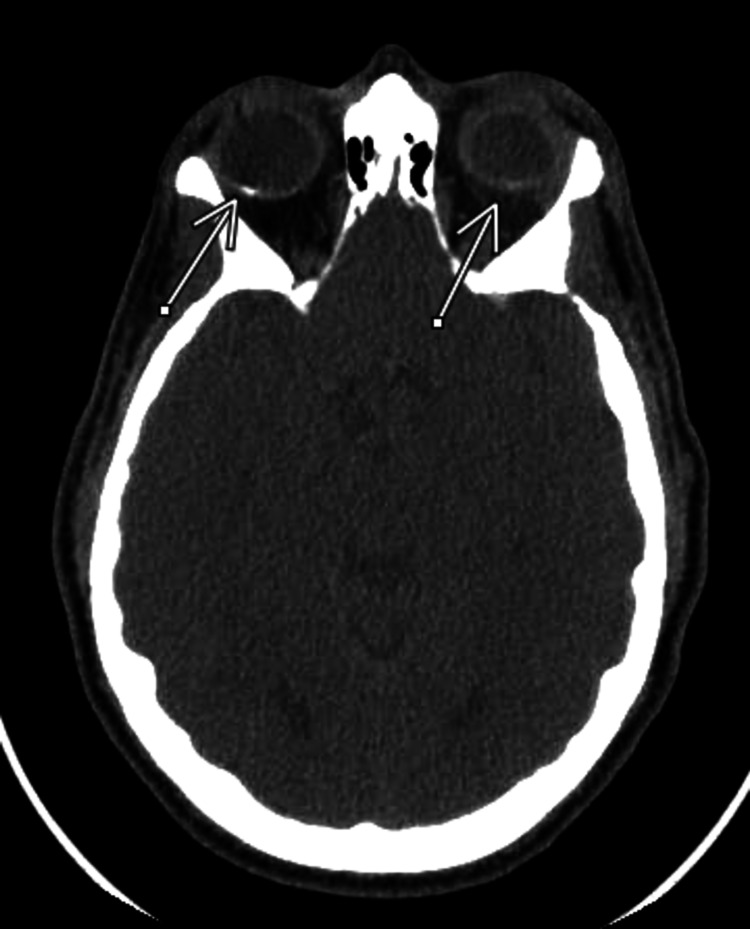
CT of the head showing retinal calcifications (arrows).
